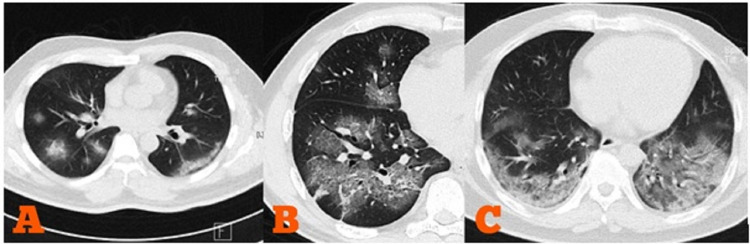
Characteristic findings of low-dose chest CT imaging in COVID-19 pneumonia found in our patients A. Multifocal and bilateral rounded GGO with predominant peripheral distribution; B. Crazy paving pattern; C. Bilateral mixed GGO and consolidation with posterobasal predominance GGO = ground-glass-opacity
